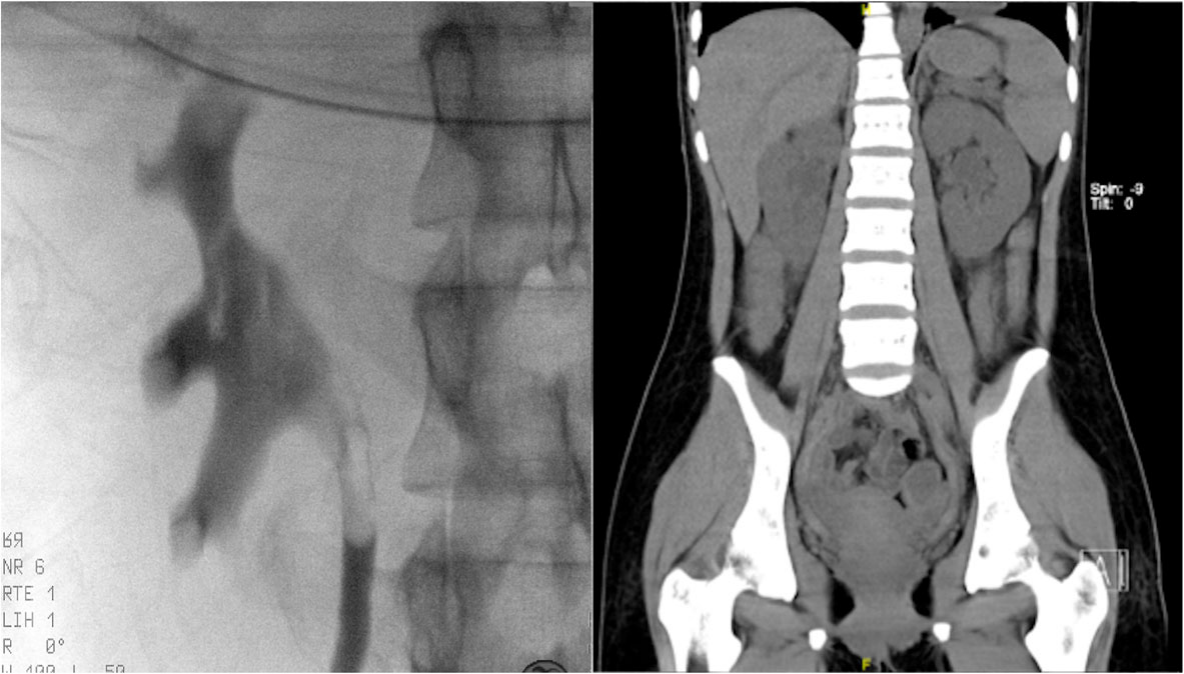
CT imaging showing bilateral hydronephrosis and mild hydro-ureters (right); fluoroscopy imaging confirming filling defect (left)
